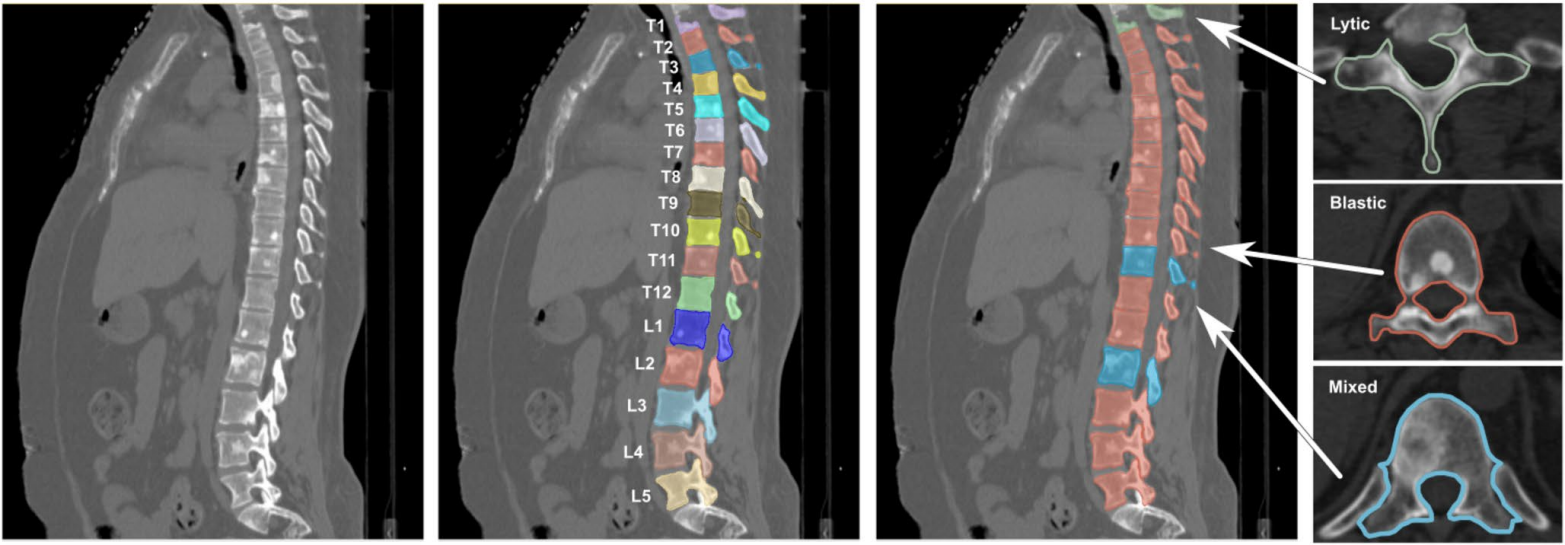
Example of artificial intelligence guided computed tomography (CT) spine segmentation and analysis of lytic, blastic, and mixed lesions. All figures labeled for reuse according to Creative Commons Non-Commercial-No-Derivatives 4.0 [53]
